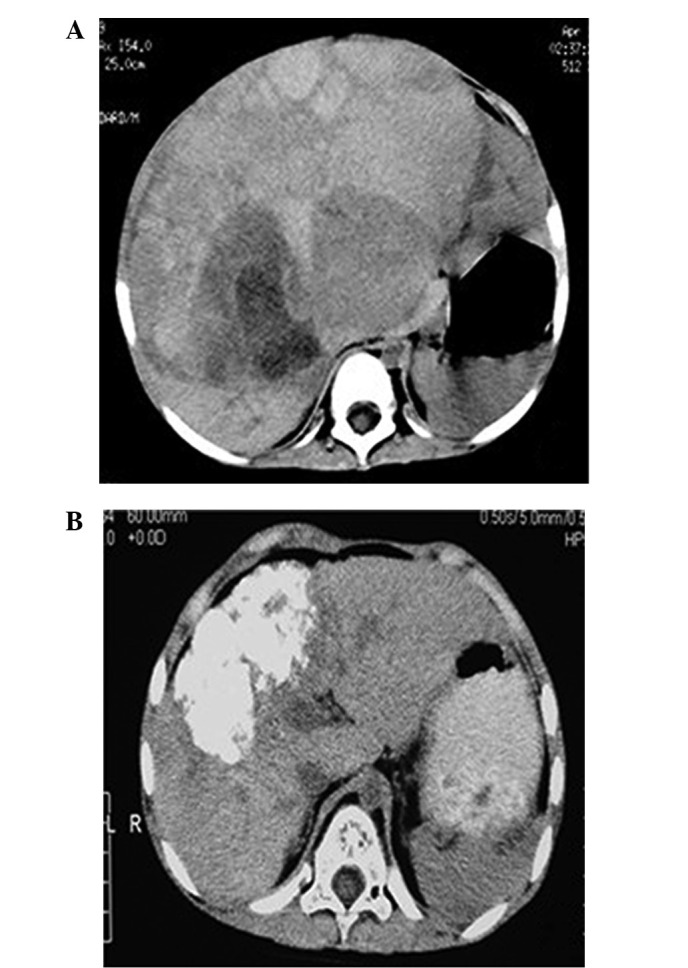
Non-contrast CT images of hepatoblastoma prior to the first TACE and following the last TACE. (A) Prior to the first TACE; CT image demonstrating a large tumor in the liver. (B) Following the last TACE; abundant lipiodol deposits appeared in the tumor, which was reduced significantly in volume. CT, computed tomography; TACE, transcatheter arterial chemoembolization.
